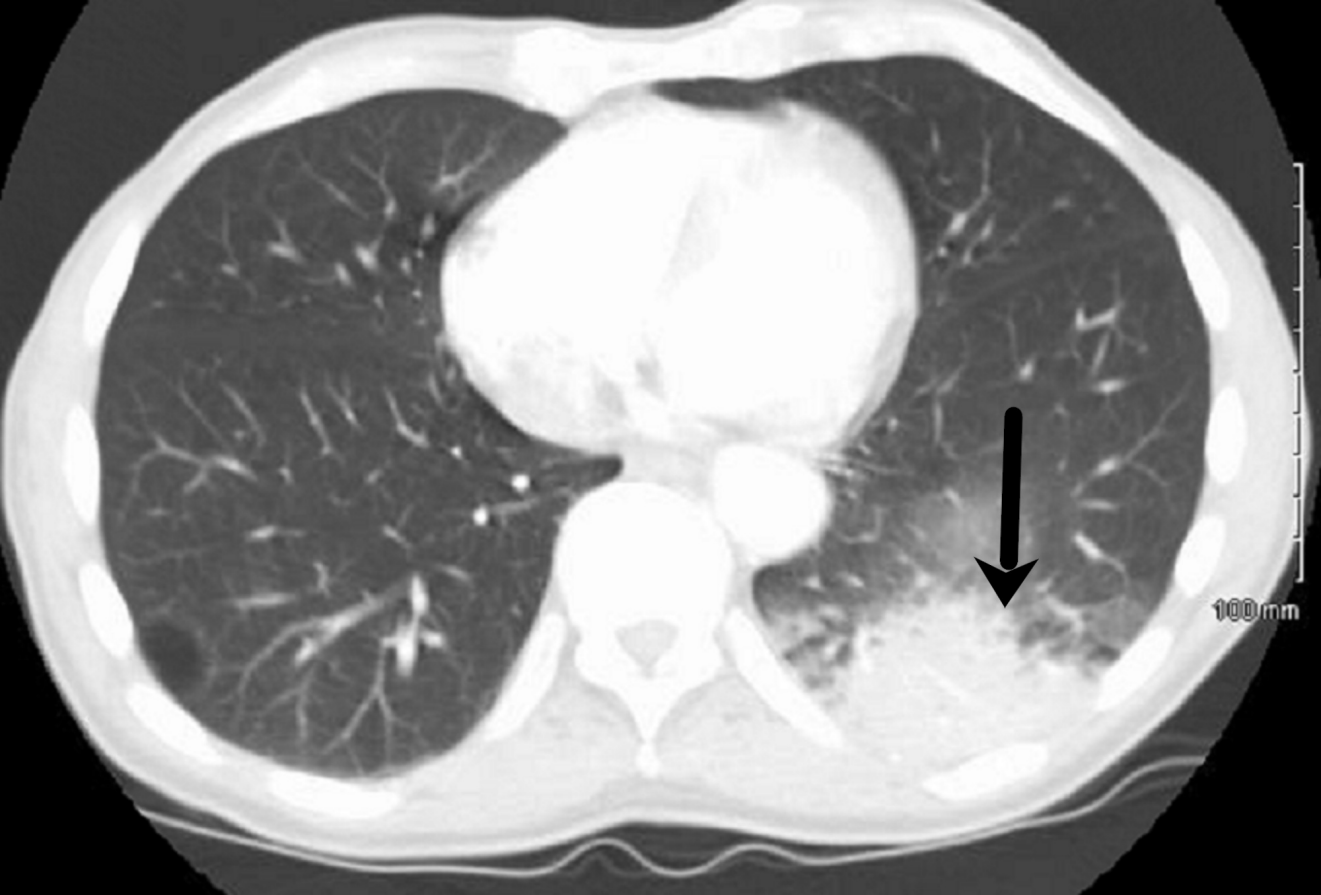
Computed tomography (CT) of the chest demonstrating a left lower lobe infiltrate
